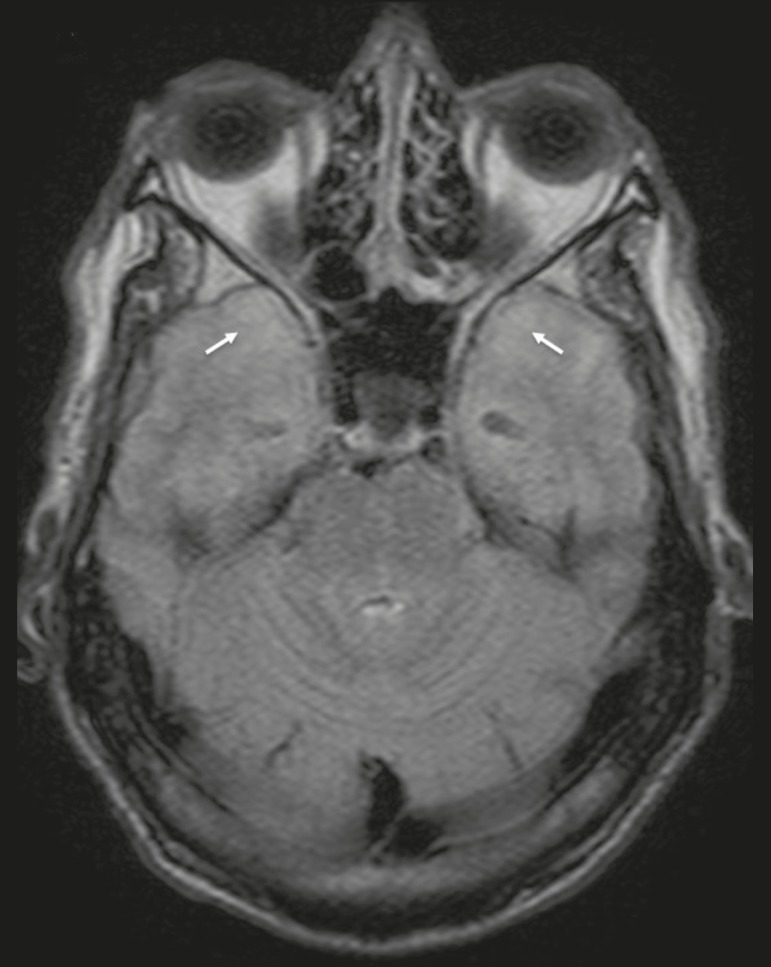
Neurosyphilis. Axial FLAIR sequence showing a hyperintense signal in the white matter of the temporal lobes.
